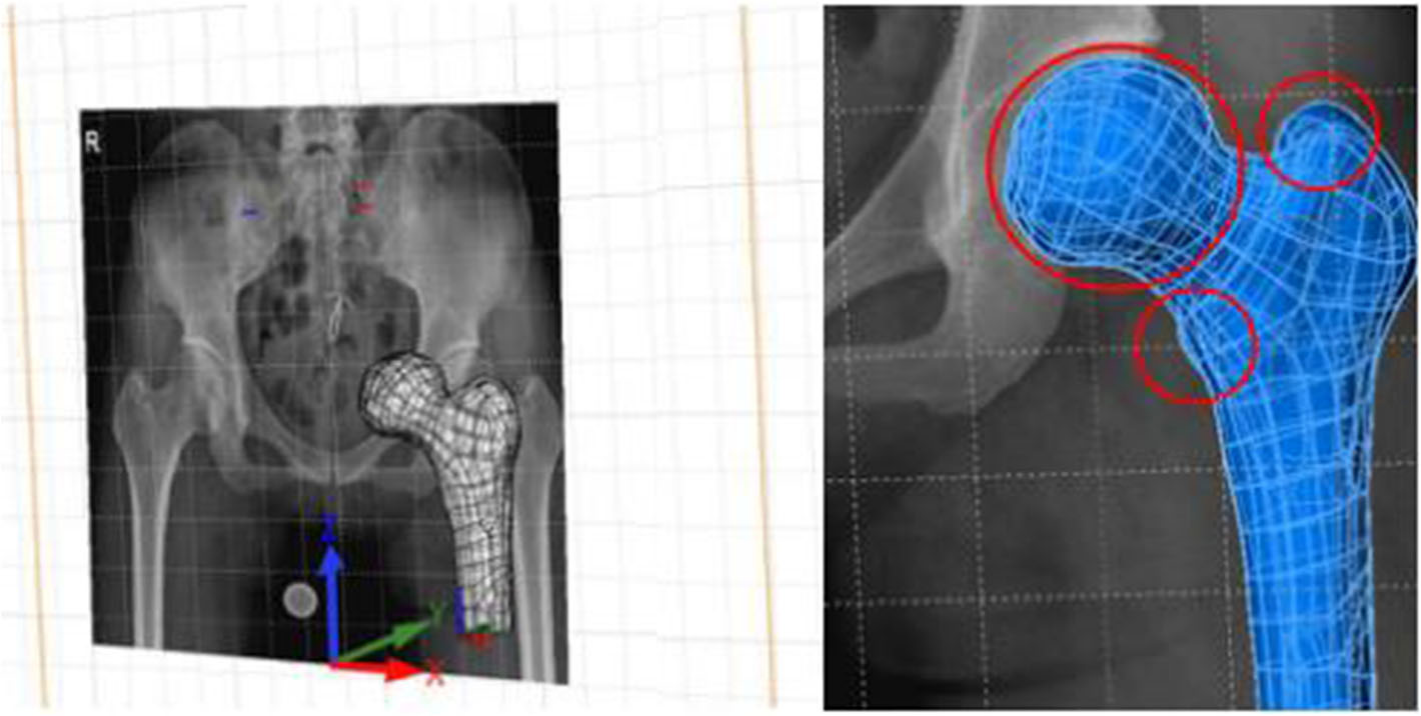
Registration of the proximal femur 3D model and X-ray image based on the greater trochanter, lesser trochanter, and femur contour
